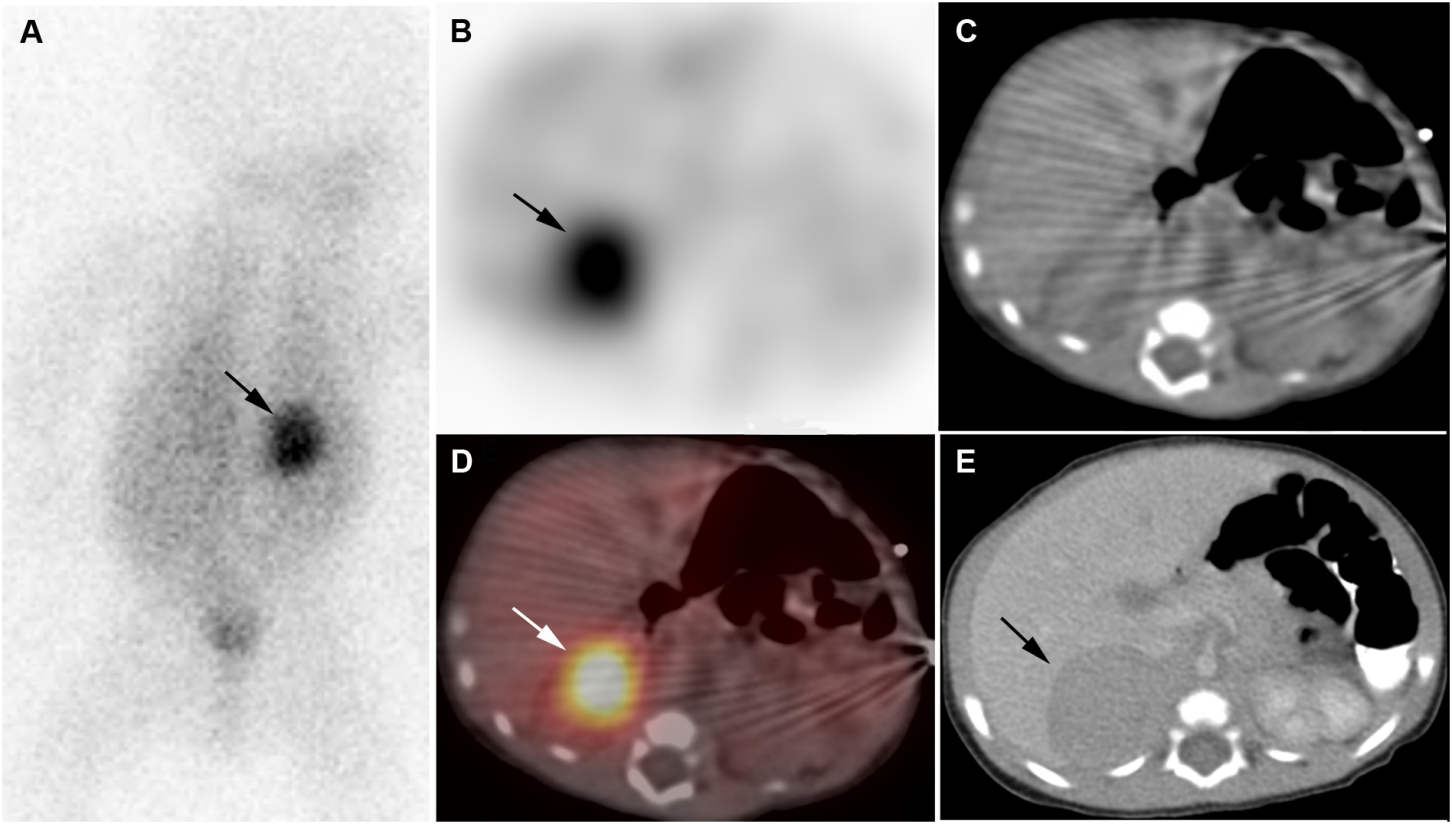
Neuroblastoma. 2-week-old boy with neuroblastoma. [^123^I]I-mIBG posterior planar image (A), [^123^I]I-mIBG SPECT transverse image (B), CT for attenuation correction (C), [^123^I]I-mIBG SPECT/CT fusion image (D), Diagnostic CT (E). Intense uptake in right adrenal mass on [^123^I]I-mIBG scan (arrows).
